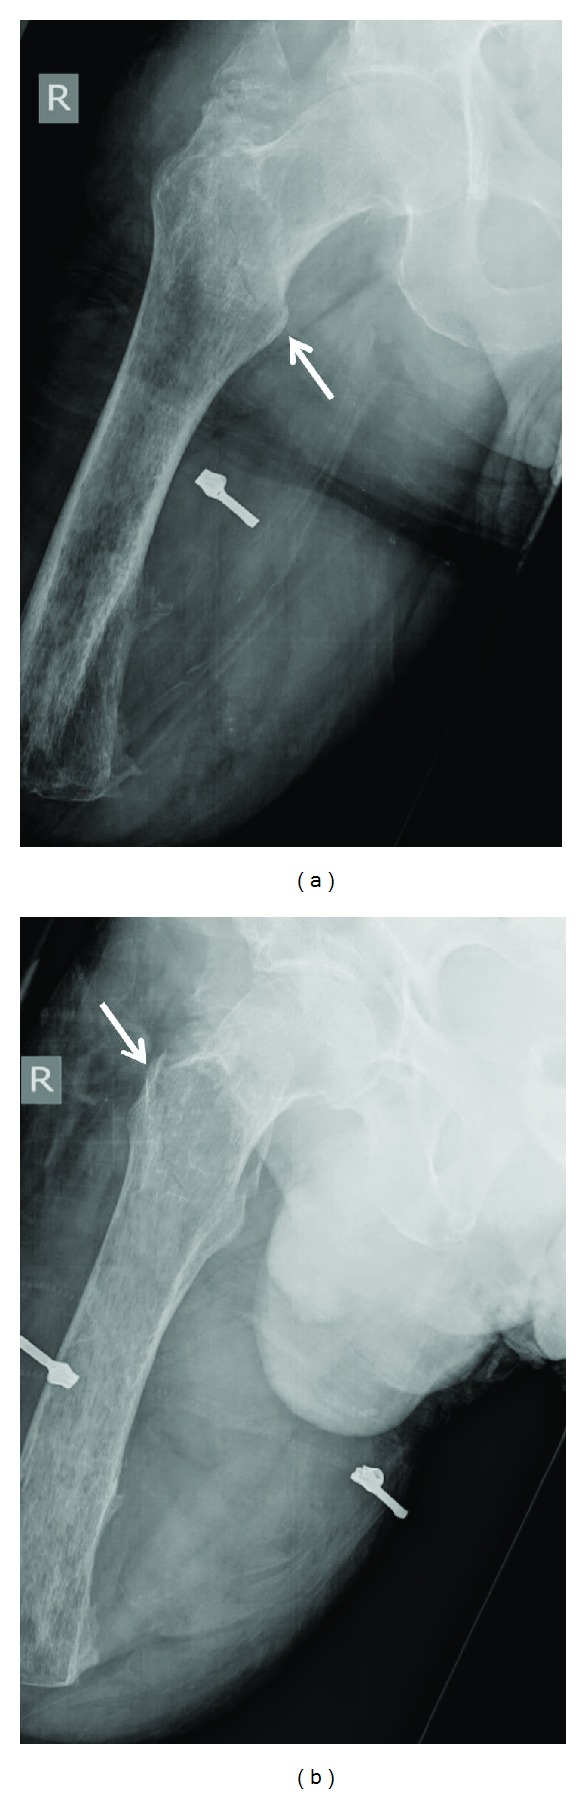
Anteroposterior and lateral radiographs of the right hip demonstrating an undisplaced extracapsular intertrochanteric fracture (white arrow).
